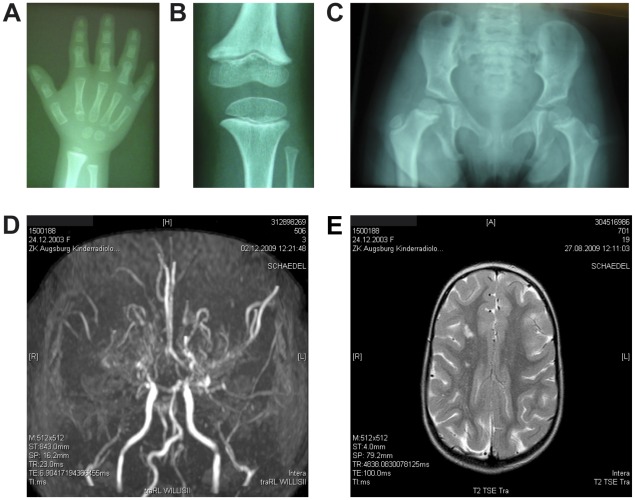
Radiological examinations and neuroimaging. A, Left hand at the age of 4.1 years: mild metaphyseal flaring of distal radius and ulna; shortening of the distal ulna; short metacarpal I; pseudoepiphyses of metacarpals II and V, shortness of the middle and distal phalanges; clinodactyly of the little finger, bone age was retarded (11 months). B, Left knee at the age of 5.7 years: V-shaped flare of the distal femoral metaphyses; triangular shape of the distal femoral epiphyses. C, Hip at the age of 5.7 years: high and narrow pelvis with broad cranial parts of the ischia; irregular metaphyses of the proximal femur; coxa vara. D, Brain MRI at the age of 5.9 years: bilateral occlusion of the intracranial internal carotid artery and the middle cerebral arteries and occlusion of the right anterior cerebral artery, prominent moyamoya collaterals; E, Brain MRI at the age of 5.7 years: old infarcts in the right white matter region.
